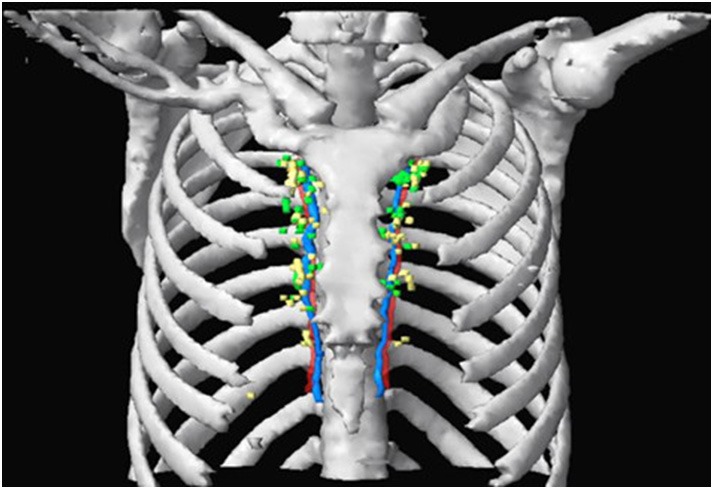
SPECT/CT fused with CT image showing IM-SLN distribution in different intercostal spaces. Green and yellow nodes indicate solitary IMNs and multiple IMNs, respectively. Red and blue indicate IM artery and IM vein.
